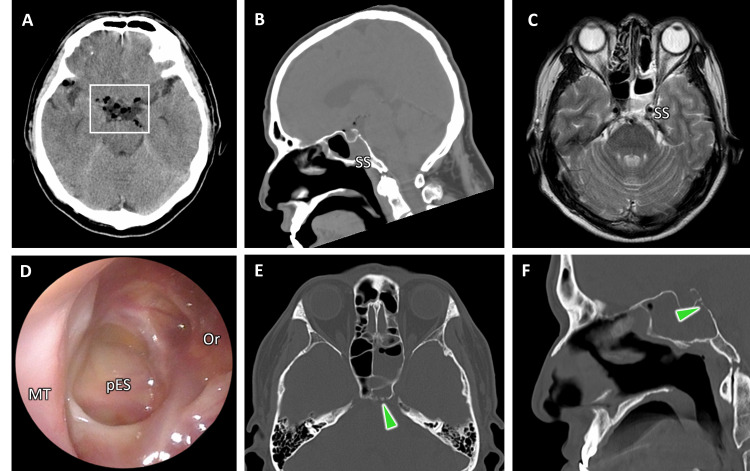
Findings at the consultation to the ENT (A) The head CT showed regions of air in the prepontine cistern (white box). (B) A partial soft tissue area was observed in the left SS. The skull base fistula was not identifiable. (C) The head MRI (T2WI) showed no mass lesion. A partial high-intensity area was observed in the left SS. (D) A pulsation and transparent viscous discharge was found in the pES. (E, F) Multiplanar reconstructed axial (E) and coronal images (F) of thin-slice CTs showed multiple bone defects (arrowheads). SS: sphenoid sinus; MT: middle turbinate or medial orbital wall; pES: posterior ethmoid sinus; T2WI: T2-weighted imaging.
